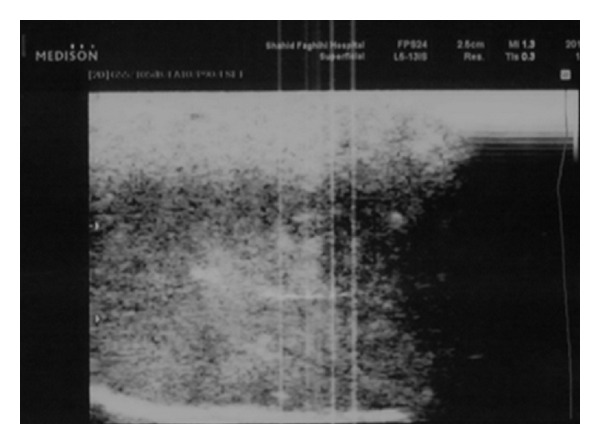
Left axillary ultrasound shows a well-defined mass with area of punctuate calcification.
